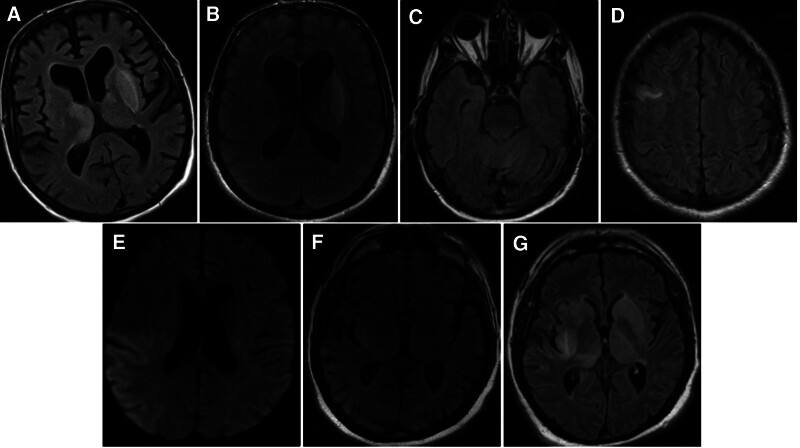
(A) Case 1: increased T_2_ weighted signal in FLAIR sequence within the left basal ganglia and medial aspect of the right thalamus. (B) Case 1: interval improvement without contrast enhancement. (C) Case 2: increased T_2_ signal on the FLAIR sequence involving the right mesial temporal lobe. (D) Case 3: linear focus of T_2_ hyperintense signal abnormality within the subcortical white matter of a single gyrus in the right frontal lobe, suggestive of cerebritis. (E) Case 4: acute infarct in the territory of the right posterior cerebral artery, specifically involving the right thalamic pulvinar. (F) Case 4: large amorphous area of faint T_2_ hyperintensity with diffusion restriction affecting the right temporal-parietal gyri, insula, sub-adjacent white matter, and basal ganglia. (G) Case 4: increased T_2_ signal in the basal ganglia and thalamic regions bilaterally, with the left side more affected than the right. FLAIR = fluid-attenuated inversion recovery.
